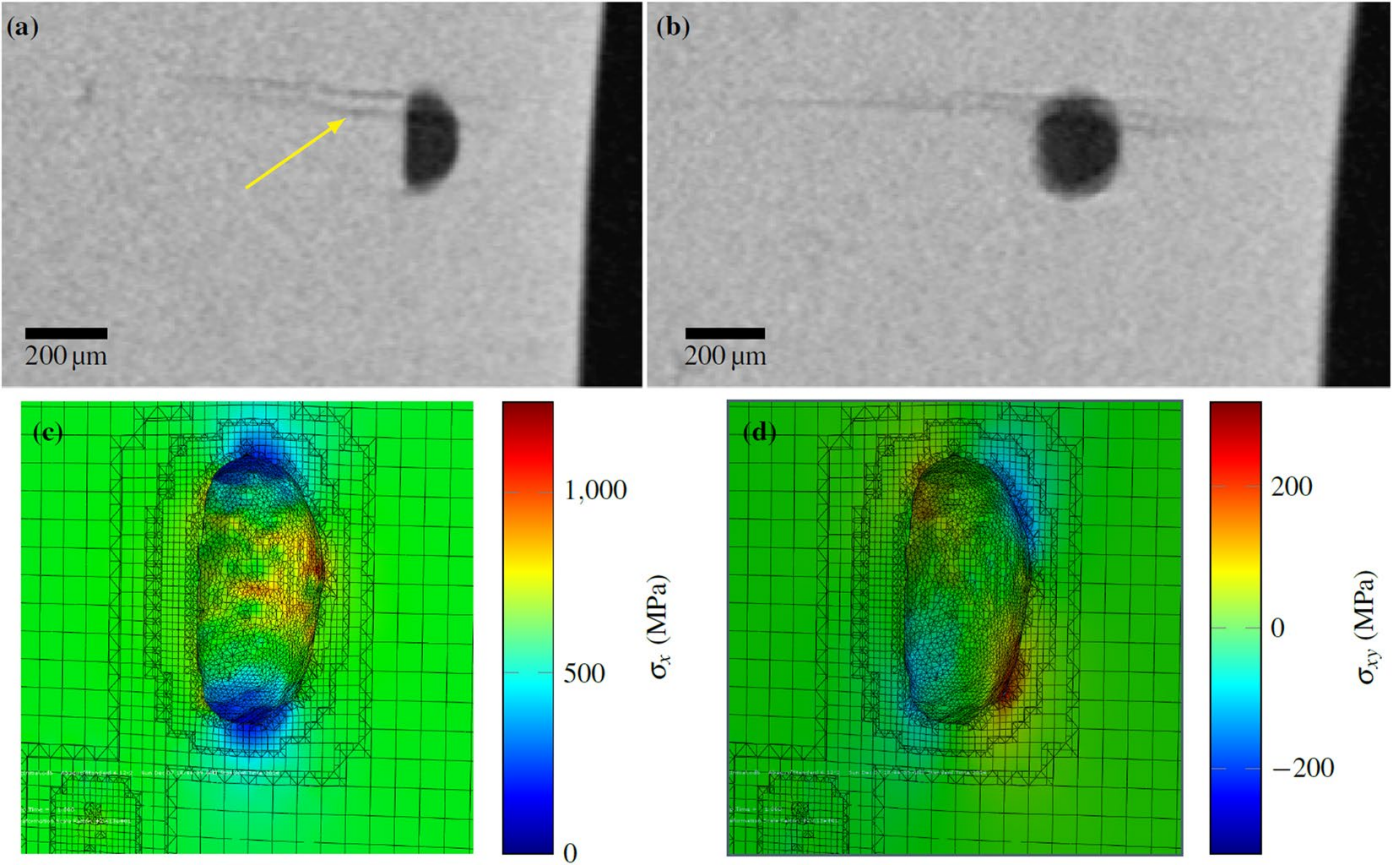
Fatigue crack initiation location in sample x-600a - Slices of the CT data in: (a) *x-y* plane and (b) *x-z* plane. Here, *x* is both the raking direction during the EBM build and loading direction during fatigue testing, while *z* is the build direction. Results of the analysis of the pore geometry detected by CT prior to testing with half the model visible, showing (c) tensile stress and (d) shear stress.
